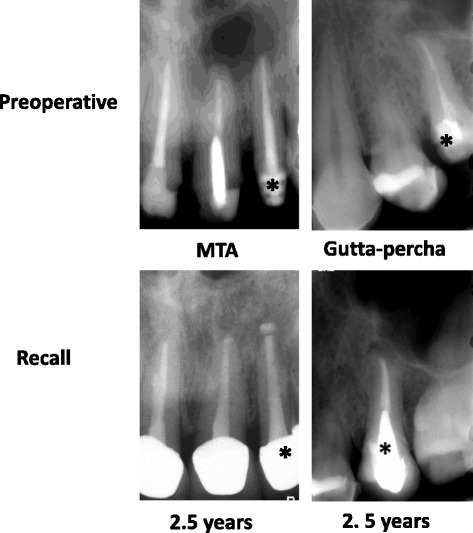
Periapical radiographs of MTA- and gutta-percha-treated teeth (*) with incomplete periapical healing at 2.5-years follow-up visits
